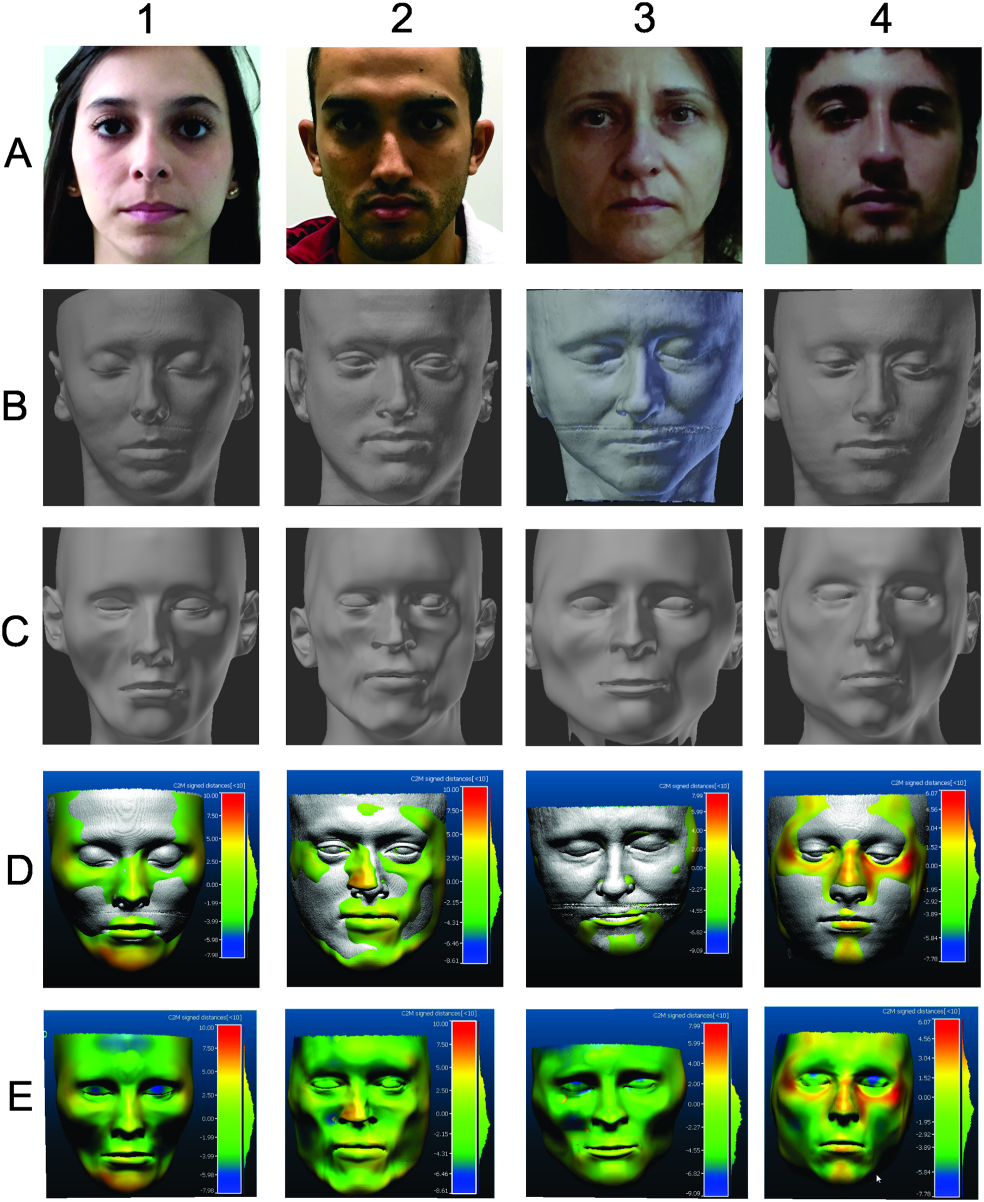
The columns show subjects 1, 2, 3, 4. Line A: photograph; line B: CT surface; line C: reconstruction; line D: result of the comparison between CT and reconstruction with the respective color map. E: reconstruction with color map.
